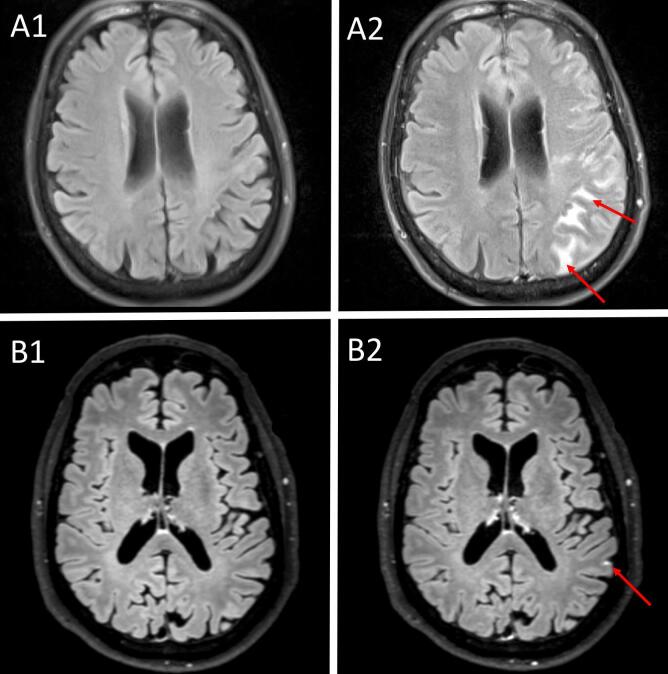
Different appearances of post-contrast T2-weighted FLAIR cerebrospinal fluid enhancement. The top row shows pre- (A1) and post- (A2) contrast T2-weighted FLAIR images in a stroke patient. The post-contrast image was acquired approximately 24 h after contrast administration and within 48 h after stroke onset, and shows widespread sulcal hyperintensities [Reprinted with permission from Springer Nature, European Radiology; license number 4818791304726 (Ostwaldt, 2015). The bottom row shows pre- (B1) and post- (B2) contrast T2-weighted FLAIR images in a cognitively normal older individual (Freeze, 2017). The post-contrast image was acquired approximately 16 min after contrast administration and shows a focal punctate hyperintensity. All images were acquired at a field strangth of 3 T. Note that the difference in appearance can possibly be attributed to individual case characteristics, but also to the difference in post-contrast acquisition timing.
